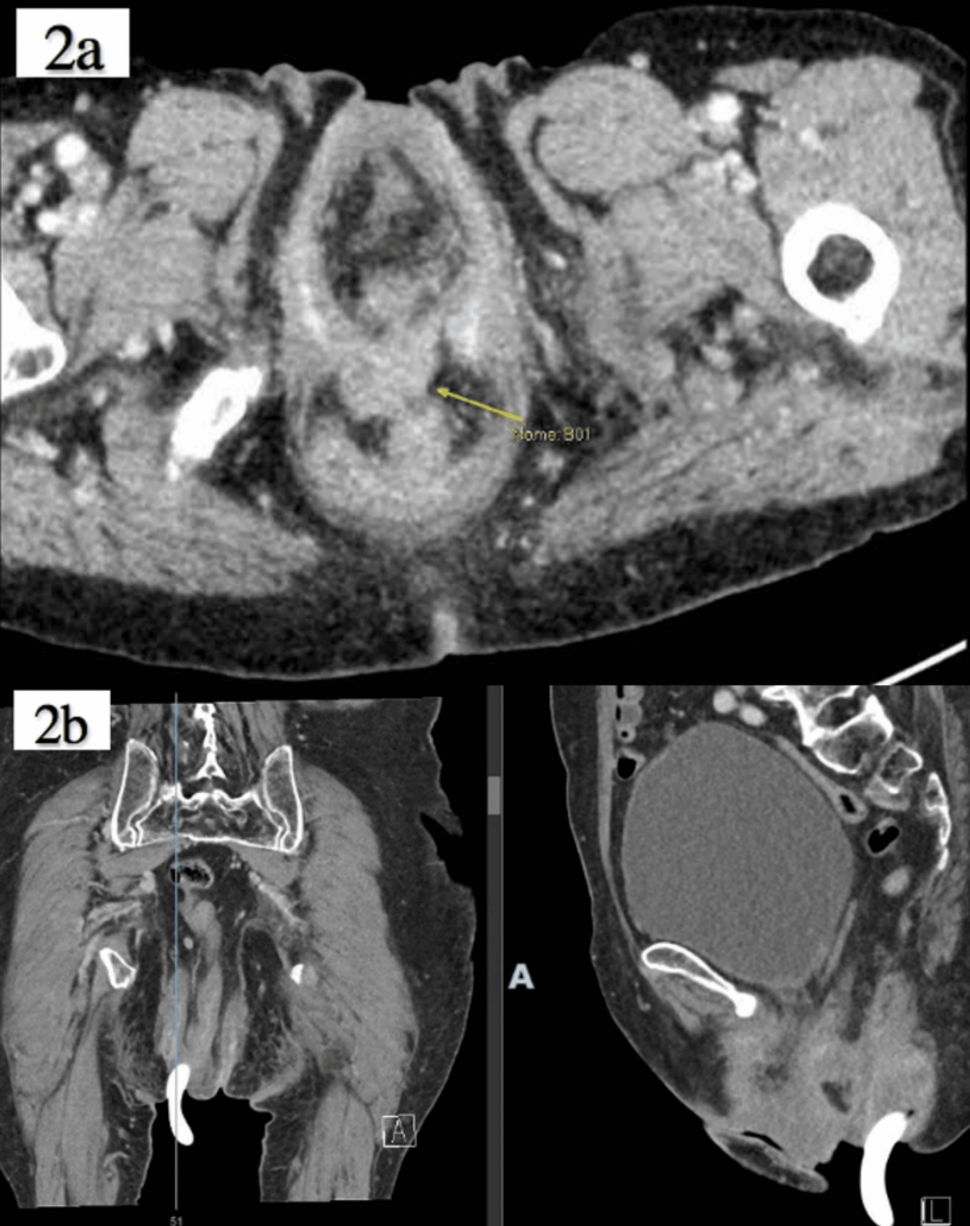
CT scan showing the chronic rectovaginal tract (a) and a pessary ring seen in prolapsed rectum: coronal view and sagittal view (b).
